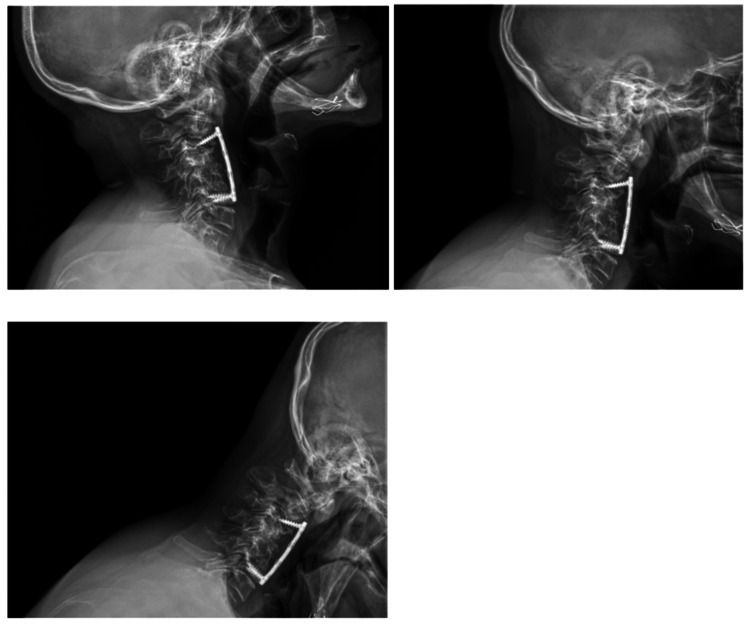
Preoperative X-ray of cervical spine
